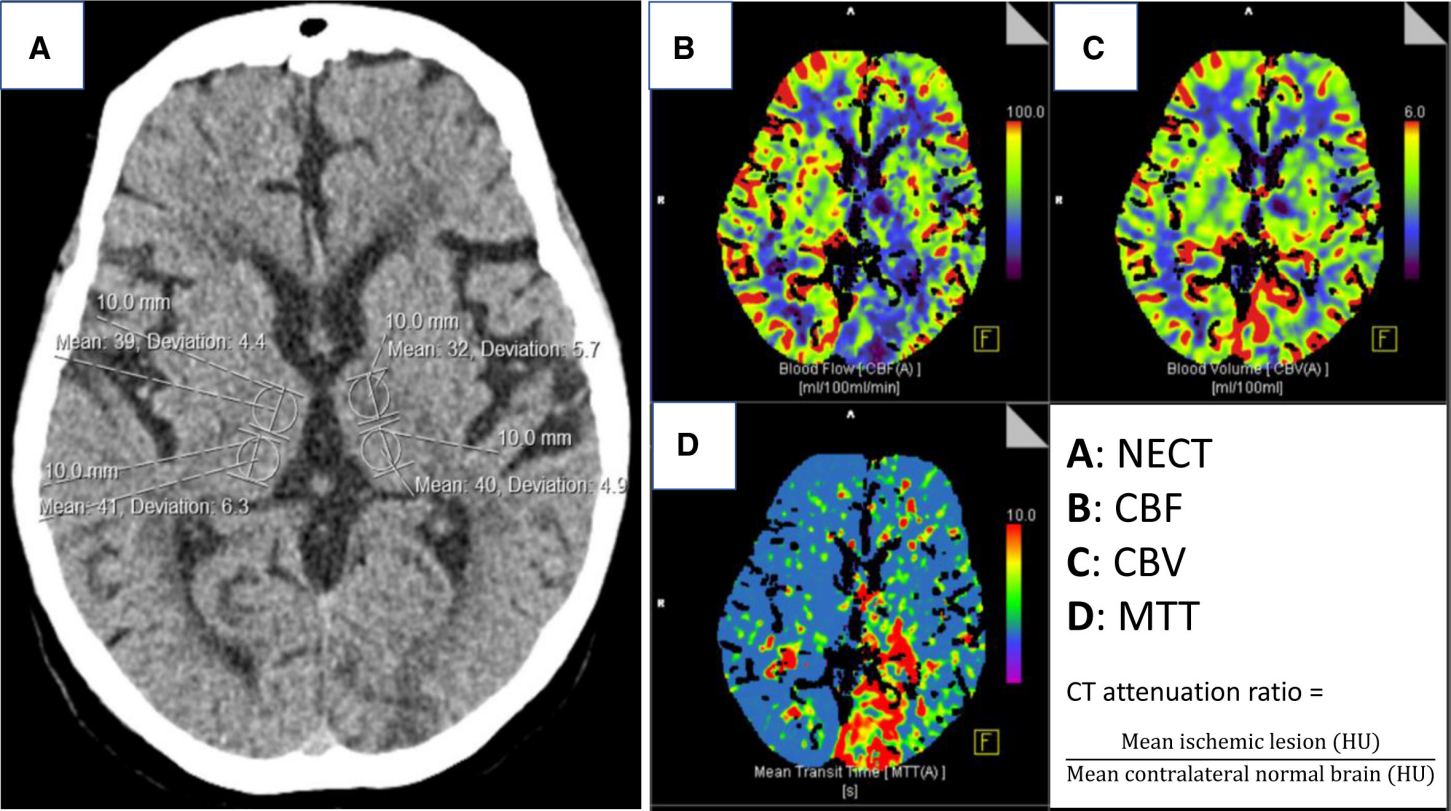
Measuring nonenhanced computed tomography (NECT) attenuation ratio for core and penumbral lesions in a 60-year-old patient with left-sided ischemia. NECT with measurements (A) and coregistered concurrently acquired computed tomography (CT) perfusion maps (B–D) demonstrate areas of lesion core (decreased cerebral blood flow [CBF; B] and cerebral blood volume (CBV; C) with increased mean transit time (MTT; D) and penumbra (decreased CBF with maintained or increased CBV and increased MTT). On NECT (A): left upper ROI measured CT attenuation of core lesion (32 Hounsfield units [HU]) mirrored to corresponding normal right hemisphere (39 HU), attenuation ratio 0.82; left lower ROI measured CT attenuation of penumbral lesion (40 HU) mirrored to corresponding normal right hemisphere (41 HU), attenuation ratio 0.98.
